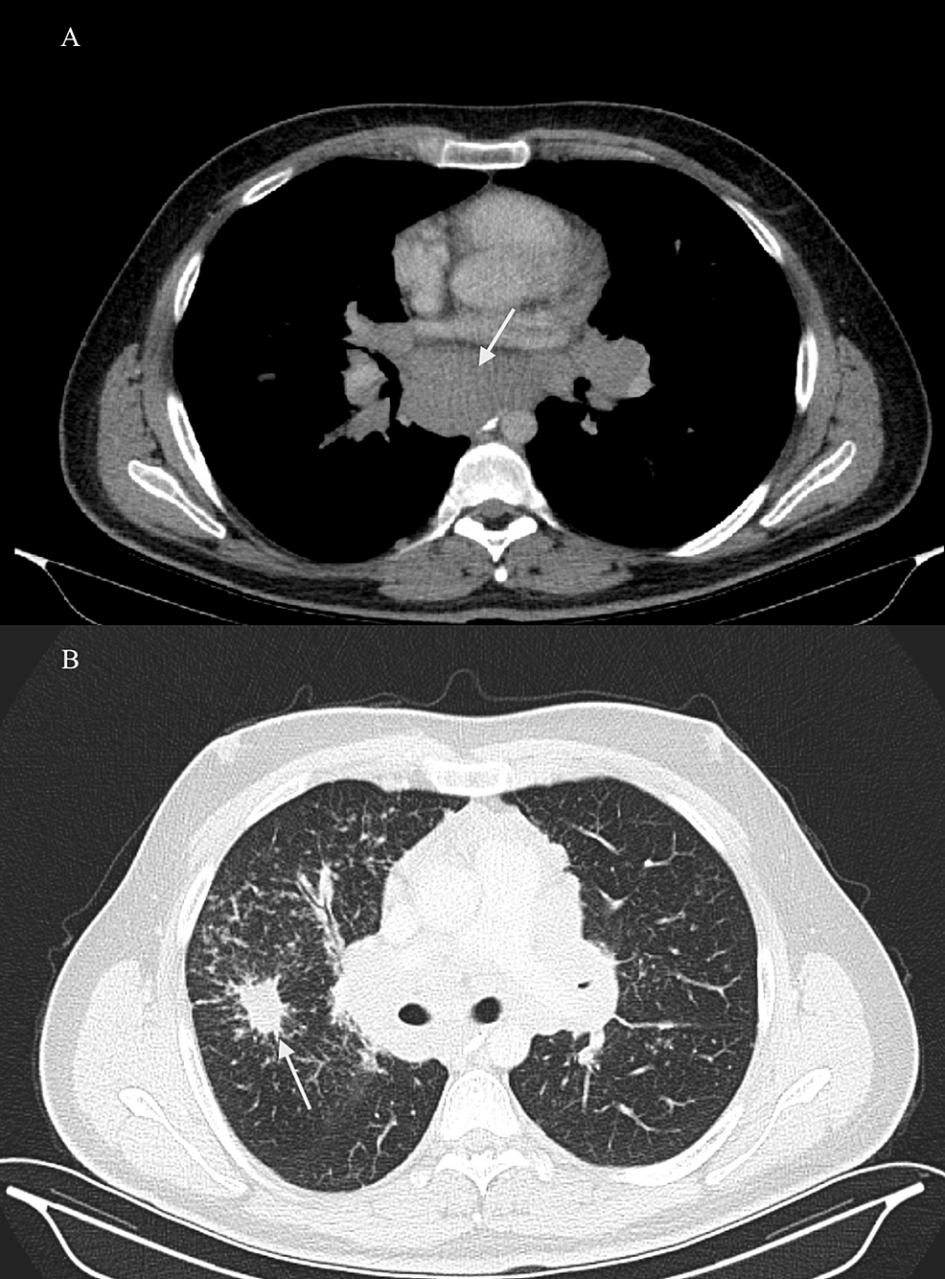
Thoracal CT shows the biggest lymph node (5x4 cm) in the mediastinum (A) and a nodule in the upper right lobe of the right lung (B) with arrows.
